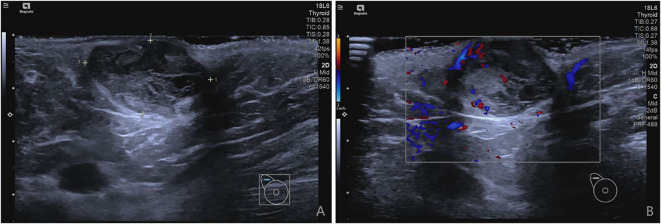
(A, B) demonstrate ultrasonographic findings of a solid hypoechoic nodule in the right axilla.
